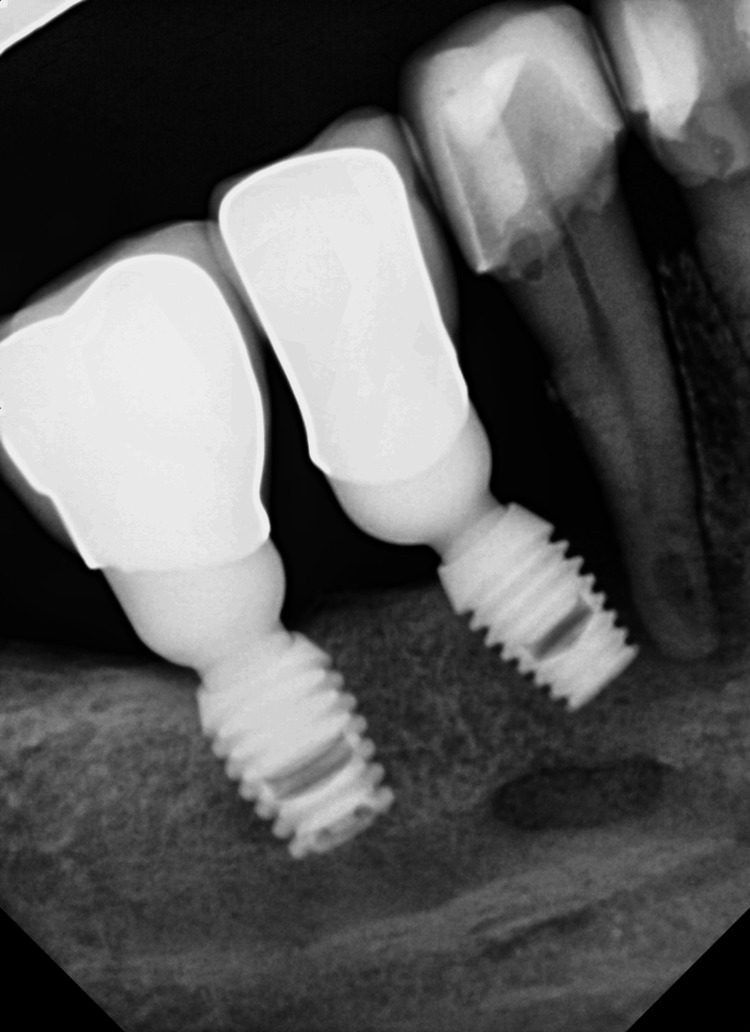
X-ray showing complete damage of septum 44/45
